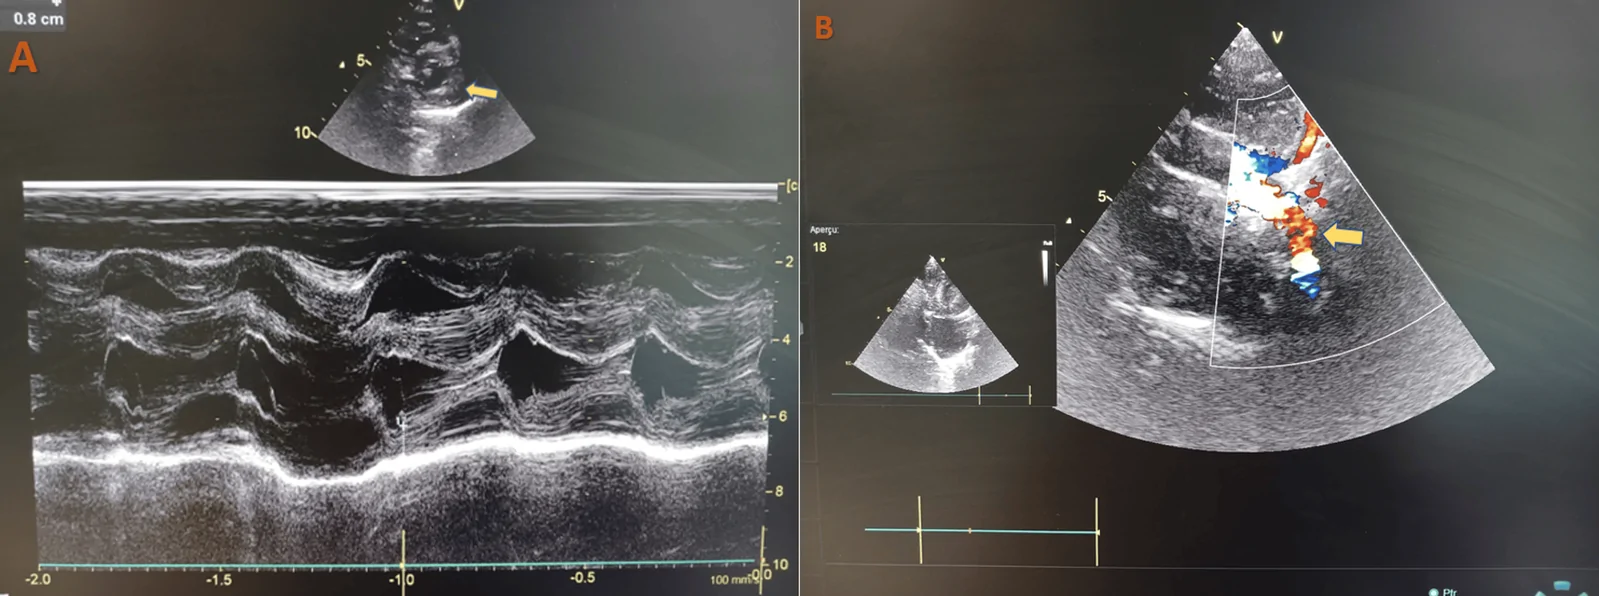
Transthoracic echocardiographic findings in a patient with RAF1-associated Noonan syndrome type 5. (A) M-mode image showing left ventricular hypertrophy (arrow). (B) Color Doppler image demonstrating mitral regurgitation (arrow).
